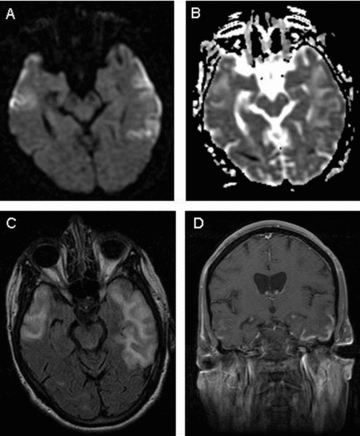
MRI brain. (A) Diffusion weighted image-revealing restriction of diffusion in gyriform pattern in bilateral temporal lobes, worse on the left, confirmed with apparent diffusion coefficient map (B). (C) FLAIR image showing hyperintesities in bilateral lateral temporal lobes. (D) Gyriform pattern of enhancement on postcontrast T1 image.
